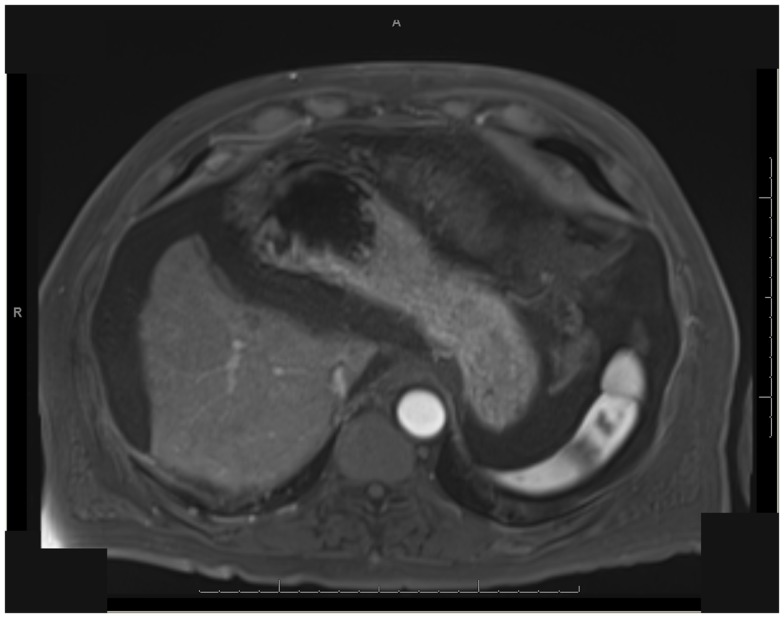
T1 MRI with early phase contrast. Status-post extended left hepatectomy, performed 6 months post-RE.
